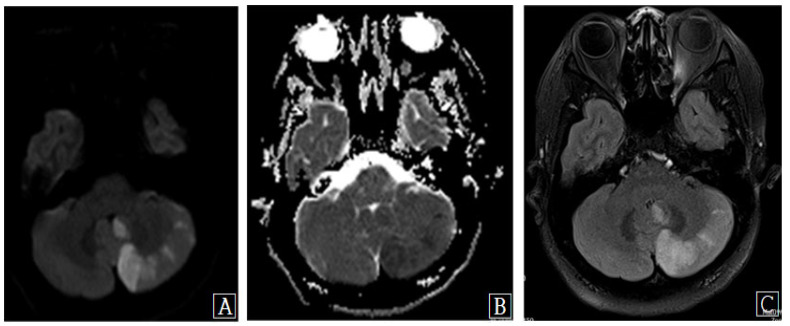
Brain magnetic resonance (MR) images show multiple patchy foci of diffusion restriction, consistent with acute left posterior inferior cerebellar artery territory infarction. (A) Diffusion-weighted MR image. (B) Apparent diffusion coefficient MR image. (C) Axial T2 flair MR image. Uneventful surgery was performed in the usual manner using a right-sided retropharyngeal approach. General anesthesia was uncomplicated, and intraoperative bleeding was well controlled, with a total blood loss of <140 mL. During the surgical procedure, the blood pressure was stable, remaining between 90 and 130 mmHg. No episodes of excessive flexion, extension, or lateral rotation occurred at any stage of the procedure. She awoke from the anesthetic neurologically intact; however, 14 h later, she complained of severe headache, dizziness, and diplopia. Urgent MRI of the cervical spine and brain was performed. MRI of the cervical spine showed satisfactory decompression of the cord and foramen; however, a large left inferior cerebellar signal abnormality indicating an acute cerebellar infarction was found on brain MRI (Figure 2).
